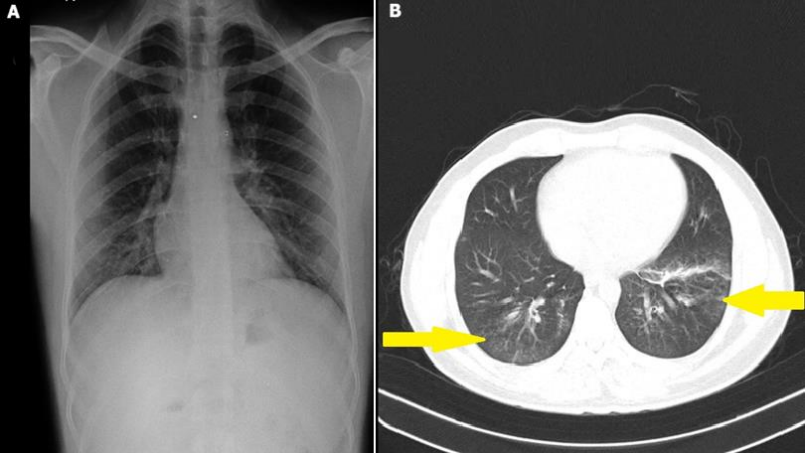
A) chest X-ray of the patient; B) computed tomography scan of the thorax showing bilateral lower lobe ground glass opacities
